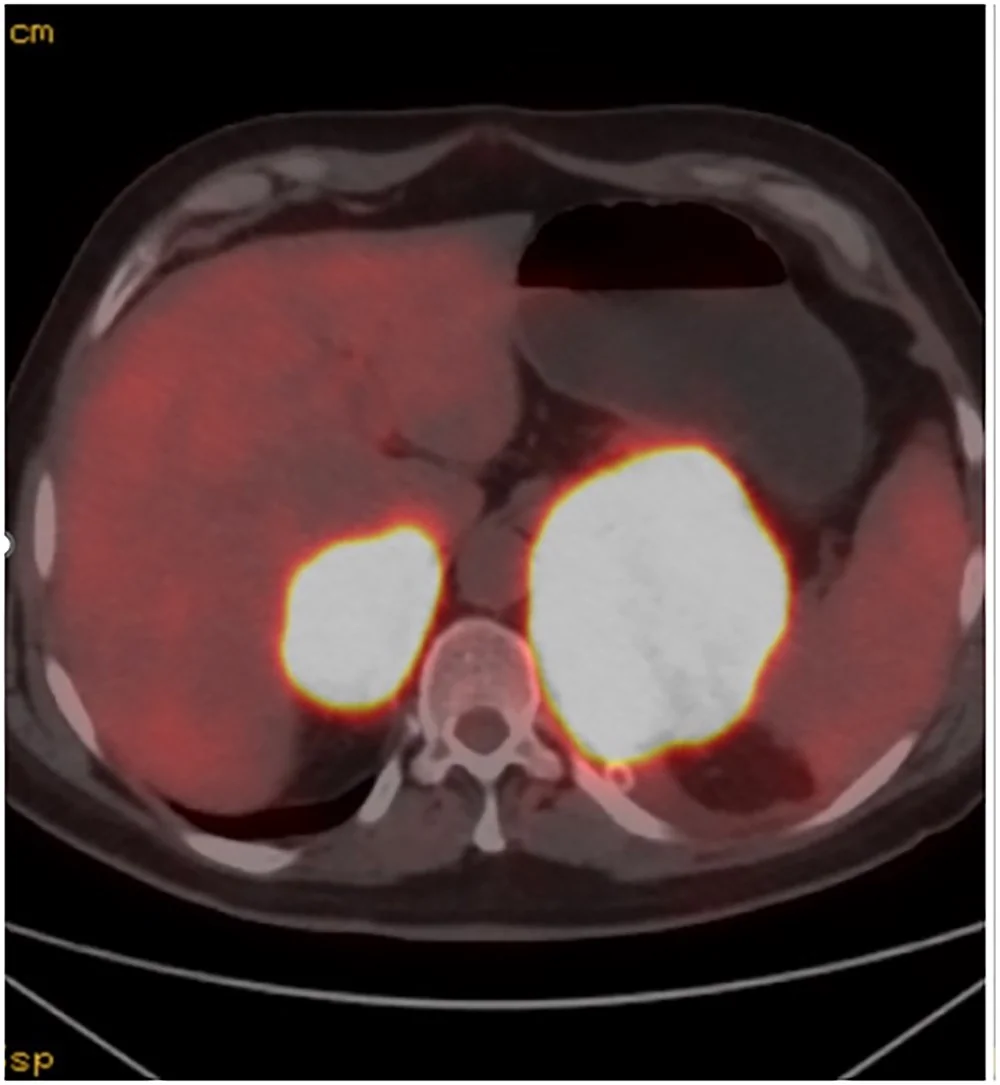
18F-FDG PET/CT fusion axial image showing increased FDG uptake in bilateral adrenal masses (SUVmax ∼ 30) and extension of the left adrenal mass into the paravertebral and retrocrural regions.
